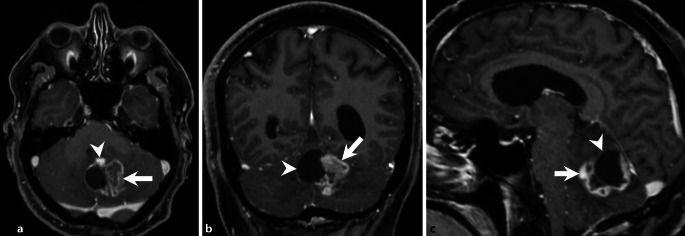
Axial (a), corona (b), and sagittal (c) T1-weighted images, acquired post-gadolinium (Gd) administration, revealed a capsular enhancement pattern in the cystic portion (a–c, arrowhead), while the solid tumor portion displayed a heterogeneous contrast enhancement (a–c, arrow)
